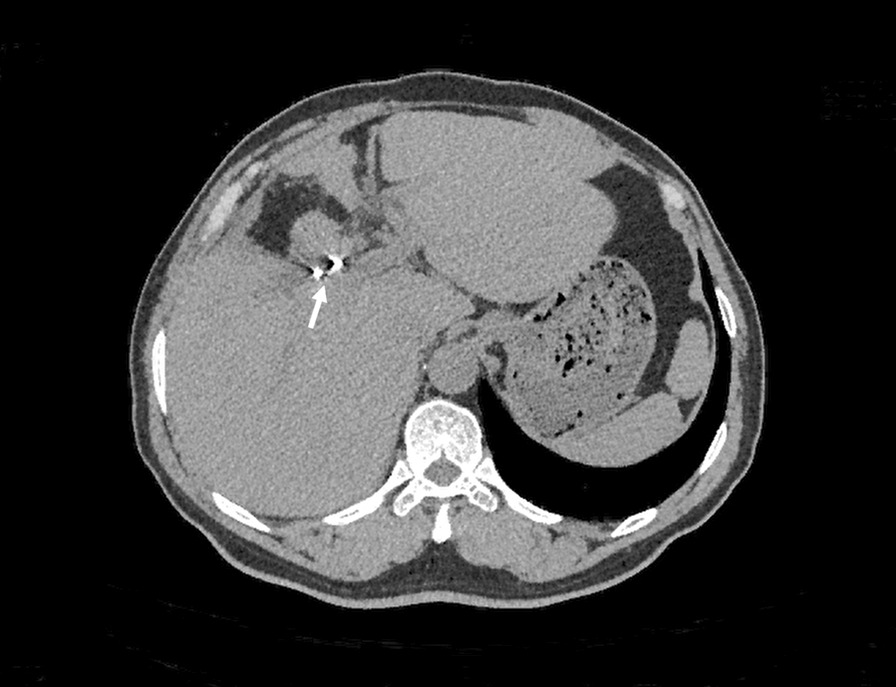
Abdominal CT scan of the last follow-up. Note the spring coil of the pseudoaneurysm
